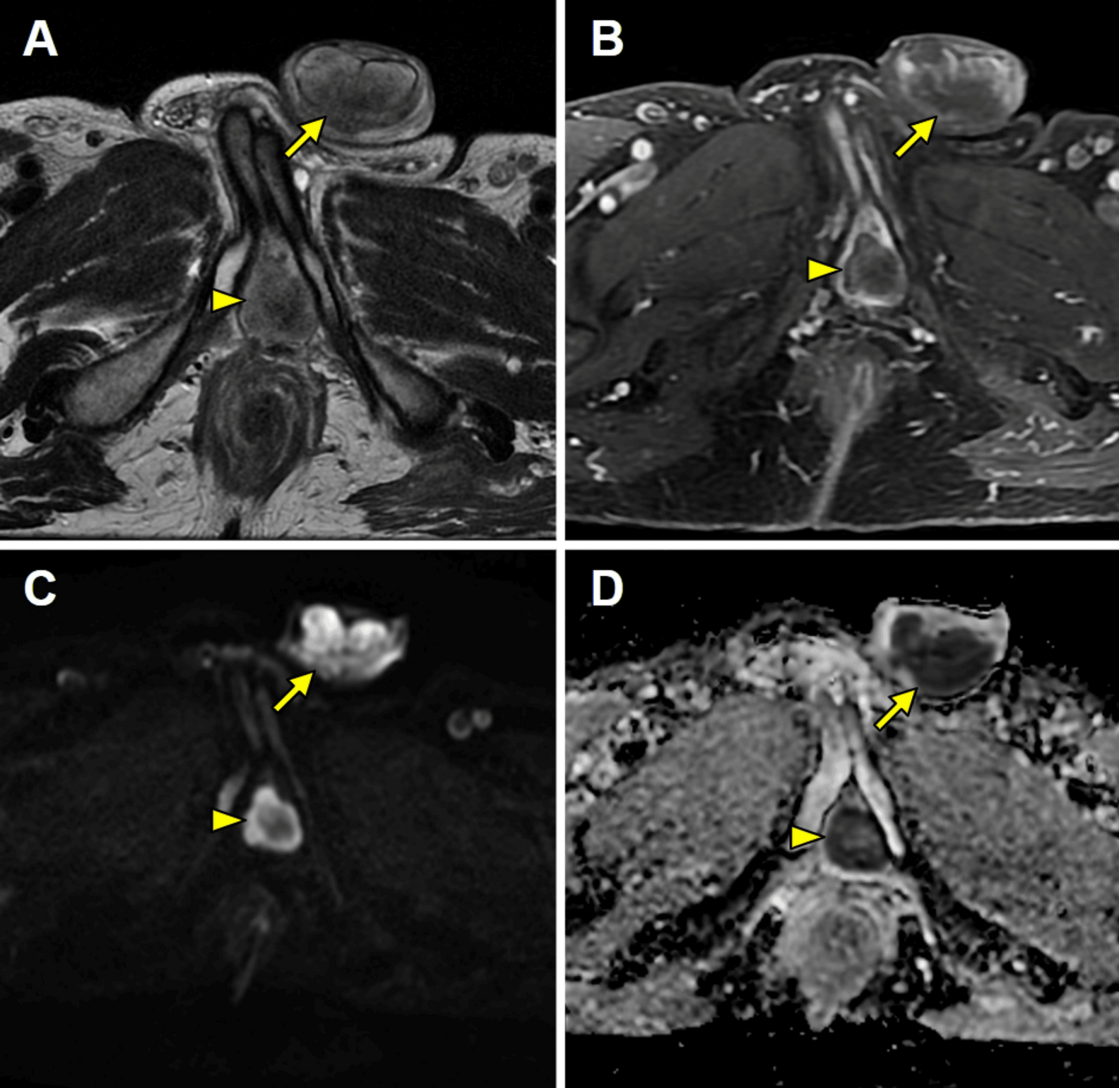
MRI study demonstrating multiple metastatic penile lesions MRI: magnetic resonance imaging Axial T2-weighted imaging (T2-WI) (A), gadolinium-enhanced fat-suppressed T1-weighted imaging (FS T1-WI) (B), diffusion-weighted imaging (DWI) (C), and apparent diffusion coefficient (ADC) map (D) reveal multiple lesions involving the corpora cavernosa and corpus spongiosum with disruption of the tunica albuginea (arrows) and the bulb of the penis (arrowheads). The lesions exhibit intermediate signal intensity on T2-WI, heterogeneous enhancement on gadolinium-enhanced FS T1-WI, and marked diffusion restriction, as indicated by high signal intensity on DWI and corresponding low signal intensity on the ADC map.
